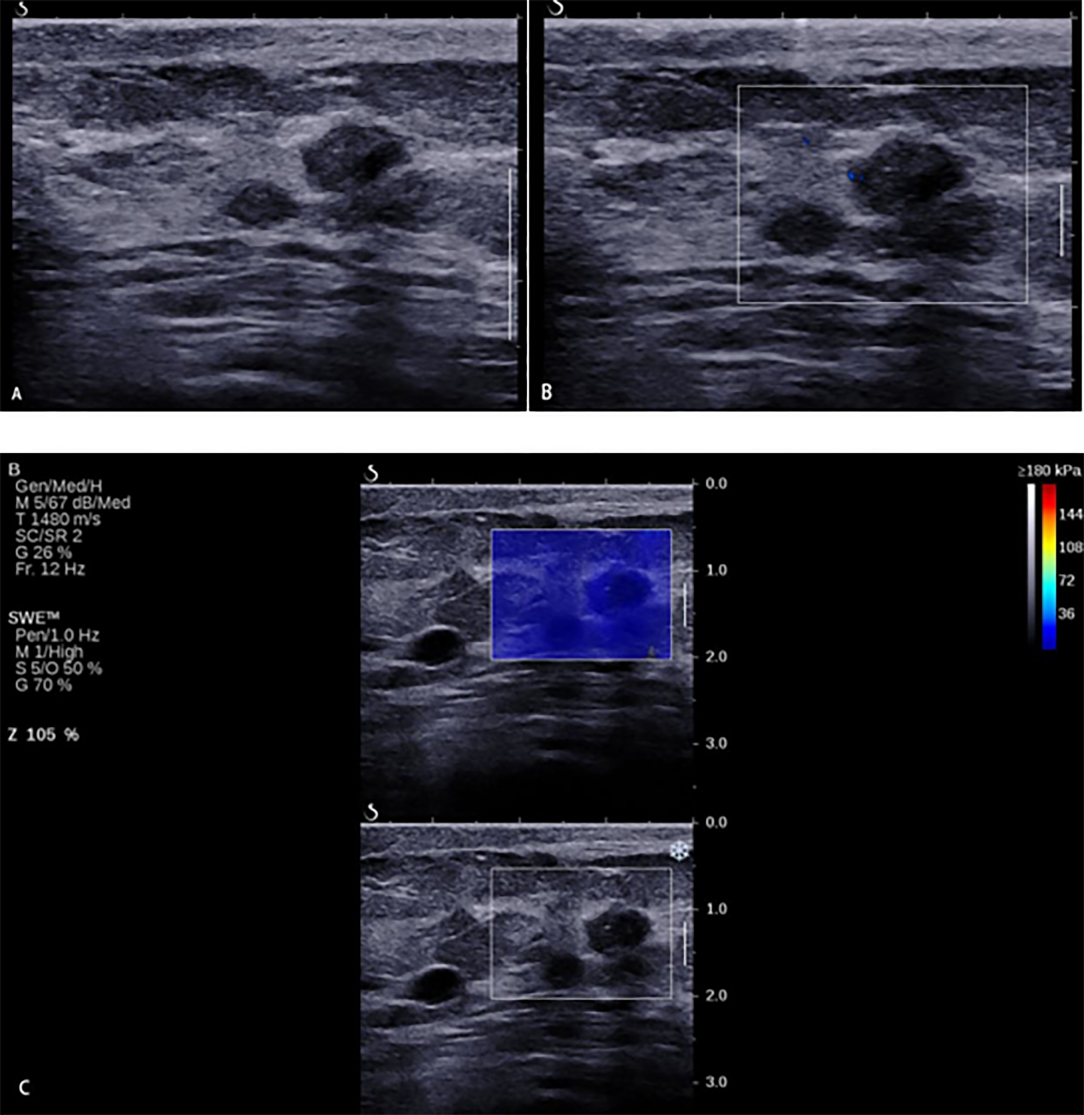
A 33-year-old woman with breast lesions. (A) The conventional B-mode ultrasound revealed an 8 × 7-mm round, hypoechoic lesion with unclear margin in the right breast, which was categorized as Breast Imaging Reporting and Data System (BI-RADS) 4a. (B) The morphologic and distribution features of the microvessels in AngioPLUS microvascular Doppler ultrasound technique followed a linear pattern. (C) The qualitative shear wave elastography (SWE) feature showed no finding. Considering the benign manifestation both in AP and qualitative SWE, the final category was downgraded into BI-RADS 3. The ultrasound-guided biopsy revealed the lesion as intermediate-grade intraductal carcinoma.
